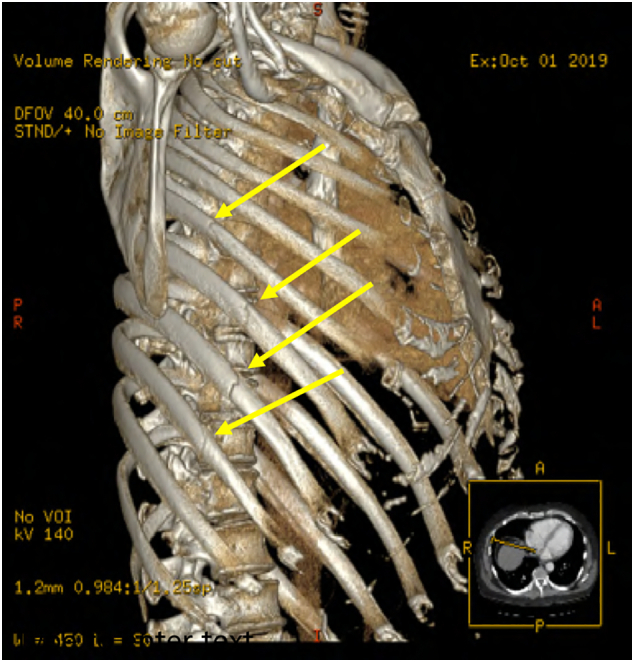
CT reconstructions demonstrating right 6th–9th rib fractures (arrows).
